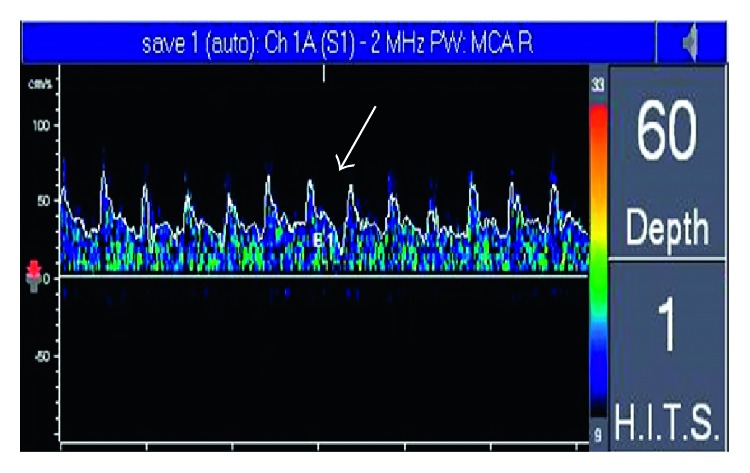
Chest radiograph at 1 hour after surgery (left) and 24 hours after surgery (right).
